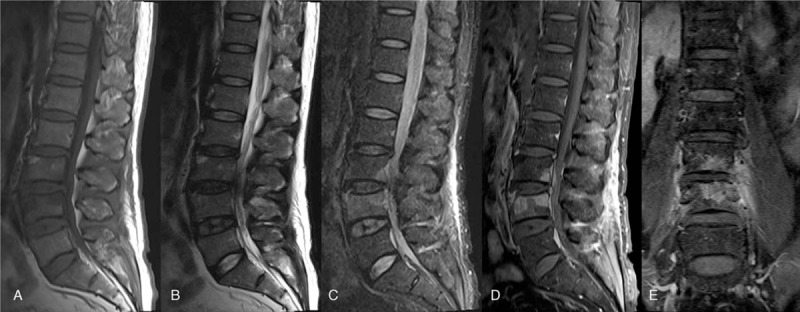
Magnetic resonance imaging findings. (A) A sagittal spin-echo T1-weighted image reveals decreased signal intensity in the bodies of the L3/4 vertebrae. (B) A T2-weighted image reveals increased signal intensity involving the L3/4 disk. (C) A fat-suppressed T2-weighted image reveals hyperintense signal intensities corresponding to the same level. (D) A sagittal gadolinium-enhanced T1-weighted image reveals areas of enhancement in the affected vertebral bodies and disks. (E) A coronal image reveals abnormally strong signals in L3/4.
